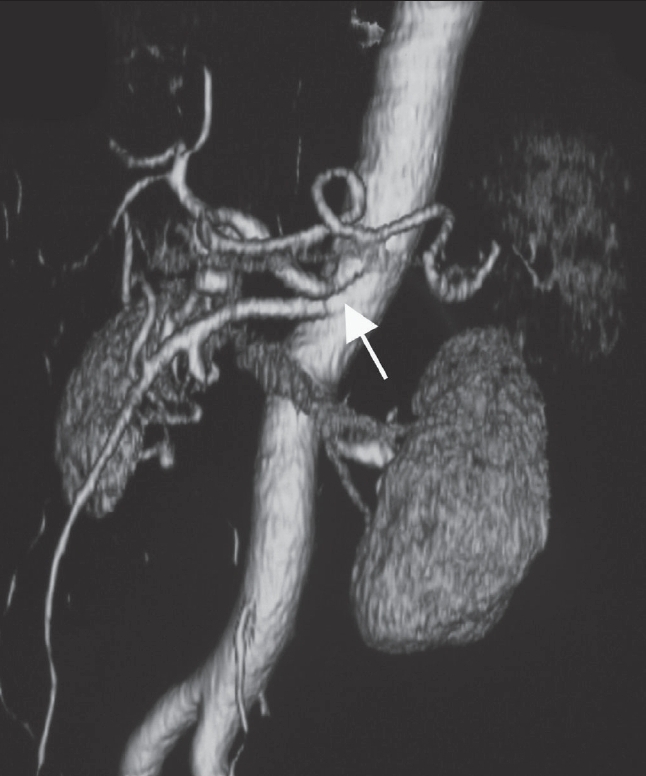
Anterolateral view of a three-dimensional CT aortogram shows stenosis of the celiac axis (arrow)
